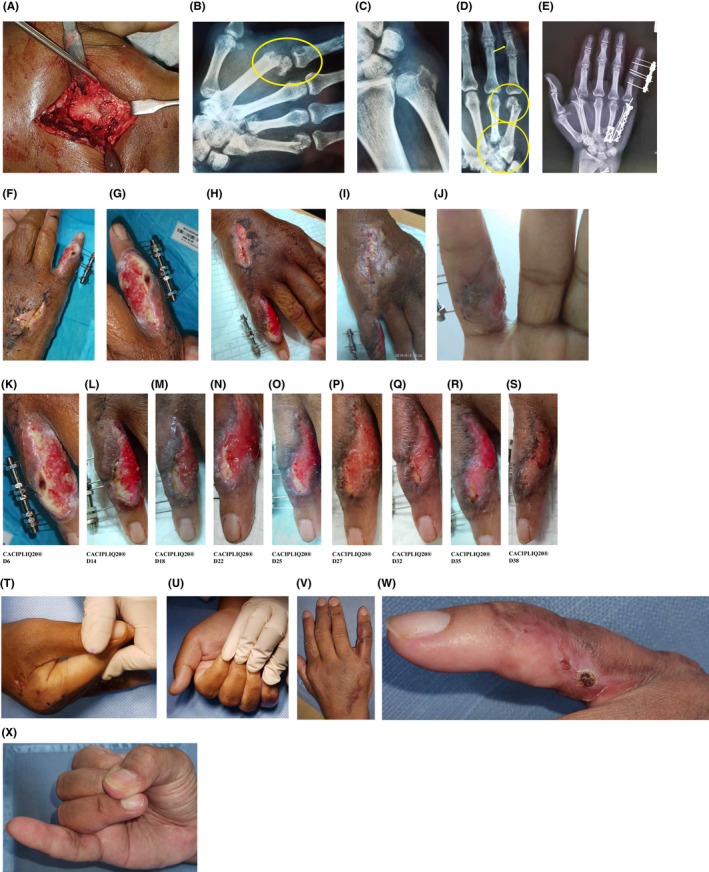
A‐D, Fixation of an open middle phalanx (P2) fracture with a concomitant degloving injury and avulsion flap of the small finger, closed 2nd and 5th metacarpal (MC) neck fractures, 4th and 5th metacarpal base fractures with dislocation of both 4th and 5th carpo‐metacarpal (CMC) joints and an Ulna styloid base fracture (yellow arrow and circles in the figures). Intraoperative fixation was prolonged and made difficult by the brittleness of the patient's bones and the lytic lesions created by the tophi. E The postoperative radiograph showed good fixation. F‐G, The wounds started to break down when blood sugar was no longer controlled. Multiple infections were found, so the dressing was changed and the wound was sprayed with Cacipliq at *POD14*. H‐I, Desloughing during dressings was performed every other day, and local application of Fucidin cream was commenced, improving the wound's status. J, Within 6 days, the wound was drier and seemed a little contracted, with healing of the volar wound as well. K‐S, The SF wound took a total of 38 days to dry up. T‐U, The patient was then taken to the theater for removal of all implants and manipulation under anesthesia. V‐X, The patient lost small finger motion but retained index finger ROM as well as its use
